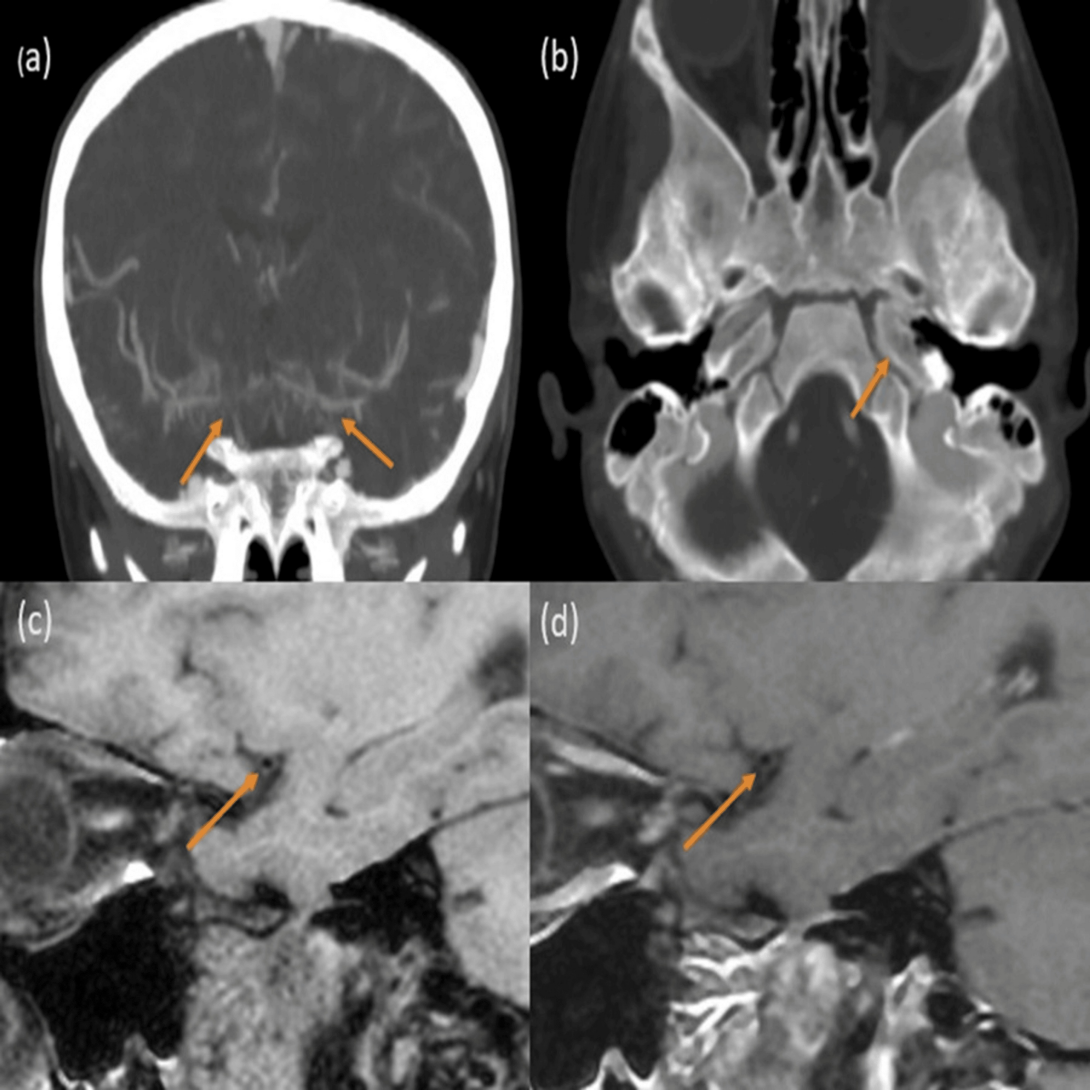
Moyamoya disease: (a) Coronal CTA images demonstrating complete occlusion of the right terminal ICA, A1 ACA, and the proximal M1 MCA and non-opacification of the left terminal ICA (agenesis) (orange arrows) with chronically developed collaterals (right>left). (b) CECT head image (bone window - agenesis of the left ICA is noted). (c and d) VW-MRI sagittal T1 BB pre- and post-contrast images demonstrating isointense circumferential non-enhancing wall thickening of the right M1 MCA (orange arrows). Diagnosis of moyamoya disease with agenesis of the left ICA is considered. CTA: computed tomography angiography; ICA: internal carotid artery; ACA: anterior cerebral artery; MCA: middle cerebral artery; VW-MRI: vessel wall magnetic resonance imaging; BB: black blood; CECT: contrast-enhanced computed tomography
